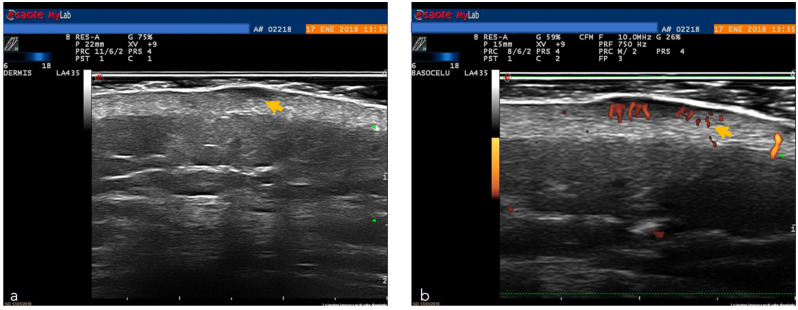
High-frequency ultrasound. A probe of 18 MHz: (a) Superficial basal cell carcinoma (yellow arrow); (b) Doppler image (yellow arrow) around the basal cell carcinoma. Image courtesy of Dr. Priscila Giavedoni.
